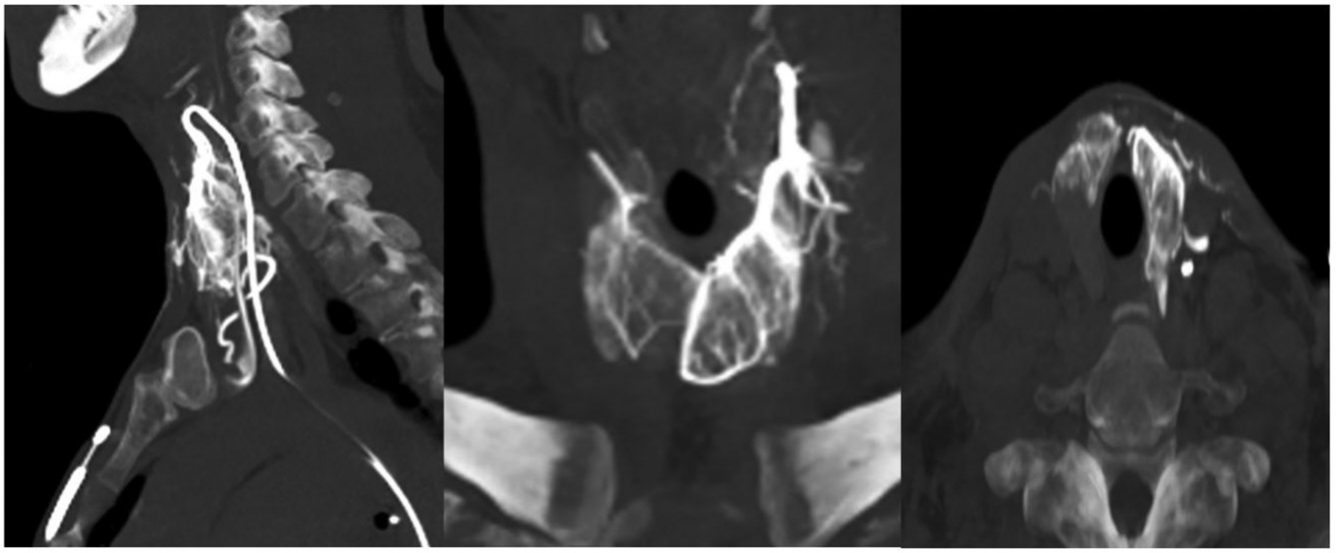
CT scan confirming left superior thyroid artery vascularization.
